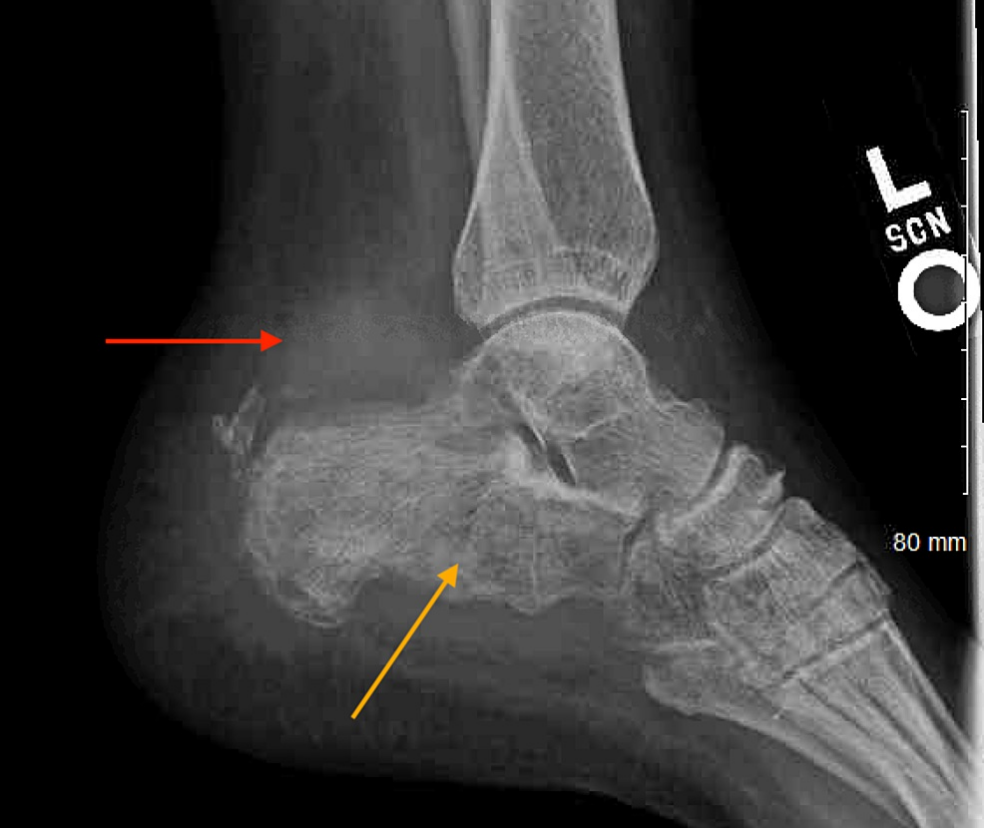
Left ankle radiograph. Left ankle radiograph showing an infiltrative soft tissue mass involving the calcaneus and adjacent posterior soft tissues. Permeative osteolysis is present (red arrow), and the calcaneus has a mottled appearance (orange arrow).
